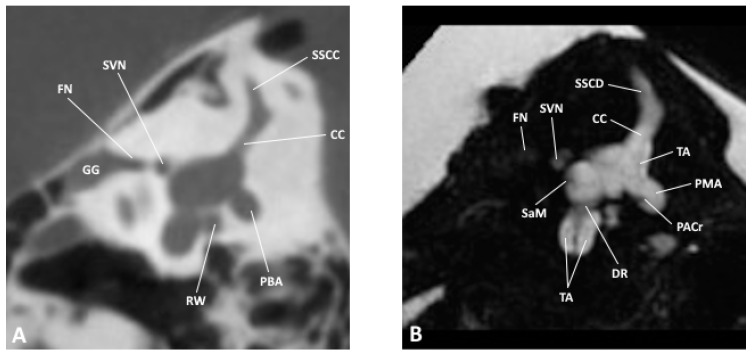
Sagittal slice through the inner and middle ear at the level of the vestibule as observed by (A) CBCT and (B) MRI; CC, common crus; DR, ductus reuniens; FN, facial nerve; GG, geniculate ganglion; PACr, posterior ampullary crest; PBA, posterior bony ampulla; PMA, posterior membranous ampulla; RW, round window; SaM, saccular macula; SSCC, superior semicircular canal; SSCD, superior semicircular duct; SVN, superior vestibular nerve.
